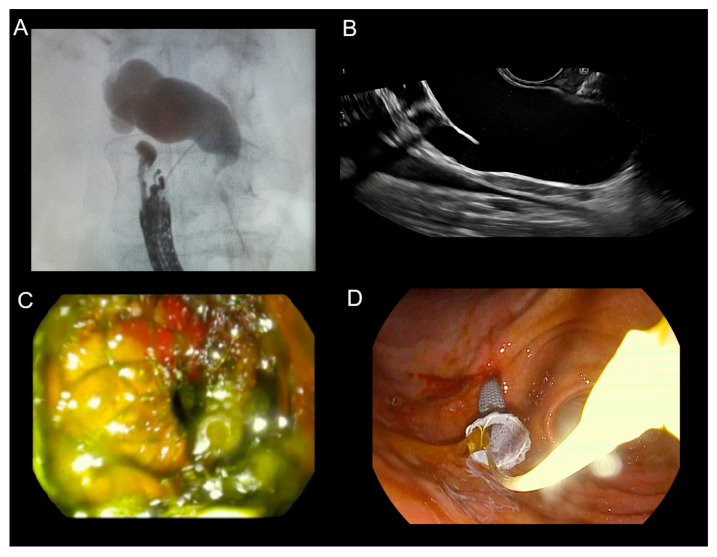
Endoscopic ultrasound-guided biliary drainage (EUS-BD) technique. (A) Fluoroscopic cholangiogram that is demonstrating a dilated common bile duct (CBD). (B) EUS view of the dilated bile duct with the puncture needle in situ. (C) Endoscopic view of a choledochoduodenostomy with a fully expanded Hot AXIOS lumen-apposing metal stent (LAMS). (D) Endoscopic view of a hepaticogastrostomy with a fully expanded Giobor LAMS.
